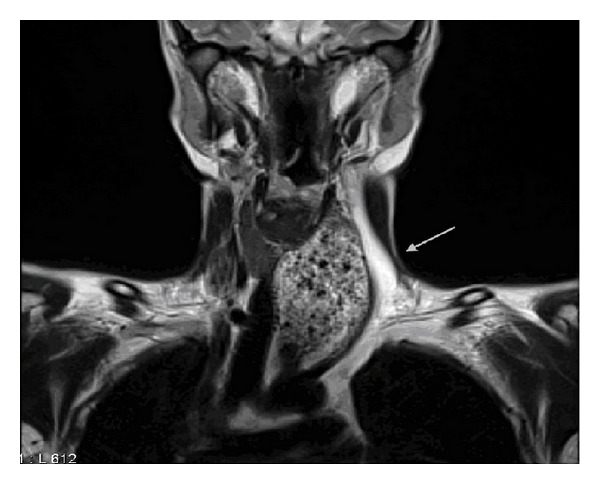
MRI scan of the neck region demonstrating a left palpable neck mass (arrow) with the presence of food remnants in the esophagus and displacement of the trachea to the right (coronal view).
